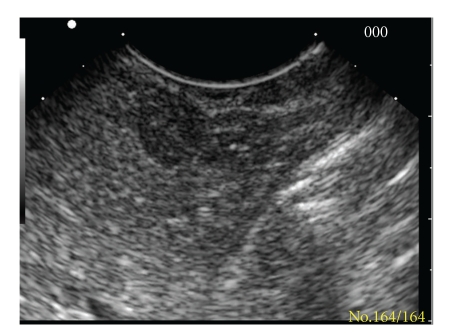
Endoscopic ultrasound of the hypoechoic lesion in the pancreatic head.
